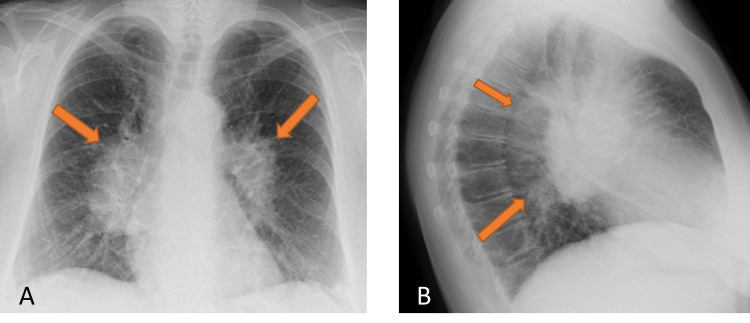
Chest X-ray showing a widened mediastinum (arrows). A. Posteroanterior view. B. Lateral view.
